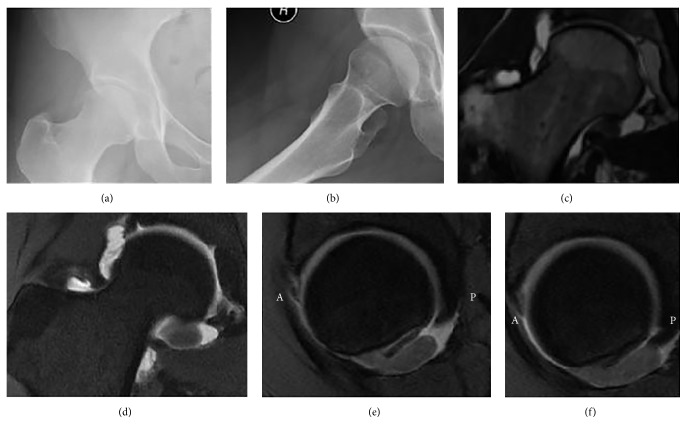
Preoperative anteroposterior (a) and frog-leg radiographs (b) of the right hip in Case 1 demonstrate developmental dysplasia of the hip and the presence of a cross-over sign. Preoperative coronal FIESTA 3D (c), coronal T1-weighted fat-saturated (d), and sagittal proton-density fat-saturated (e, f) magnetic resonance sequences with intra-articular contrast reveal the tumor, a 2.5 cm intermediate to low signal focus within the inferomedial aspect of the right hip joint, in addition to a complex tear of the right anterior and anterosuperior acetabular labrum. A = anterior; P = posterior.
